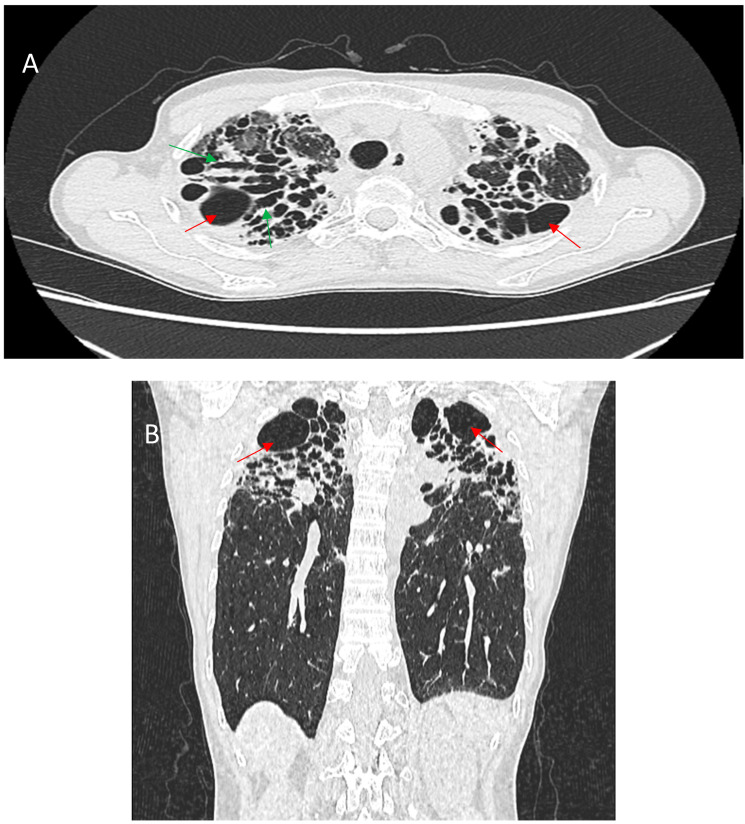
Enhanced axial CT scan (A) and coronal reconstruction (B) show a prominent typical pattern of fibrosis. There is severe upper zone volume loss associated with architectural distortion, cystic spaces (red arrows), and traction bronchiectasis (green arrows).
